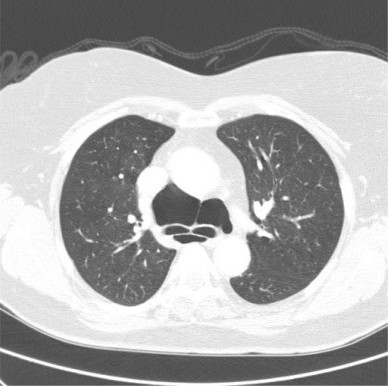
Contrast enhanced axial CT-scan of the thorax showing the air-filled cystic mass both anterior and posterior to the carina (lung window setting).
